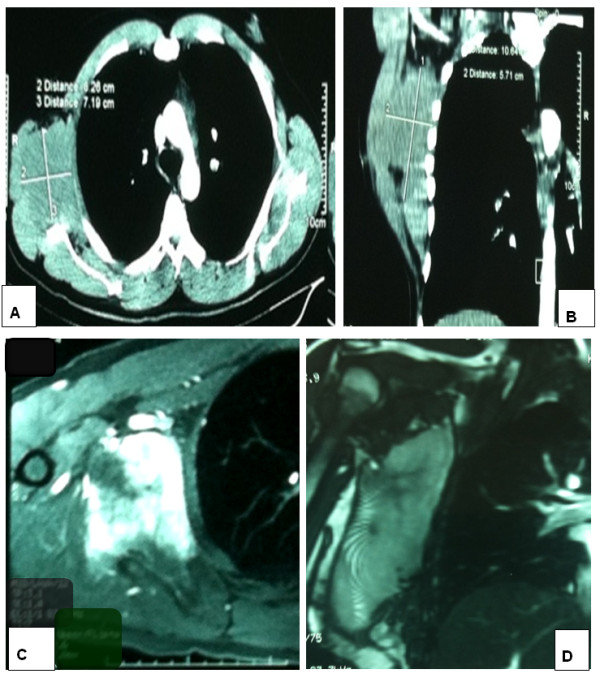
Images of the first recurrence. Thoracic computed tomography axial cut (A) and sagittal cut (B). Auxiliary magnetic resonance imaging with axial (C) and sagittal (D) cut showing mass measuring 130mm. This mass was localized on the right chest wall, between scapula and chest wall, hypointensity T1, hypersignal T2; this mass displaces the muscle structures; auxiliary vessels are permeable.
